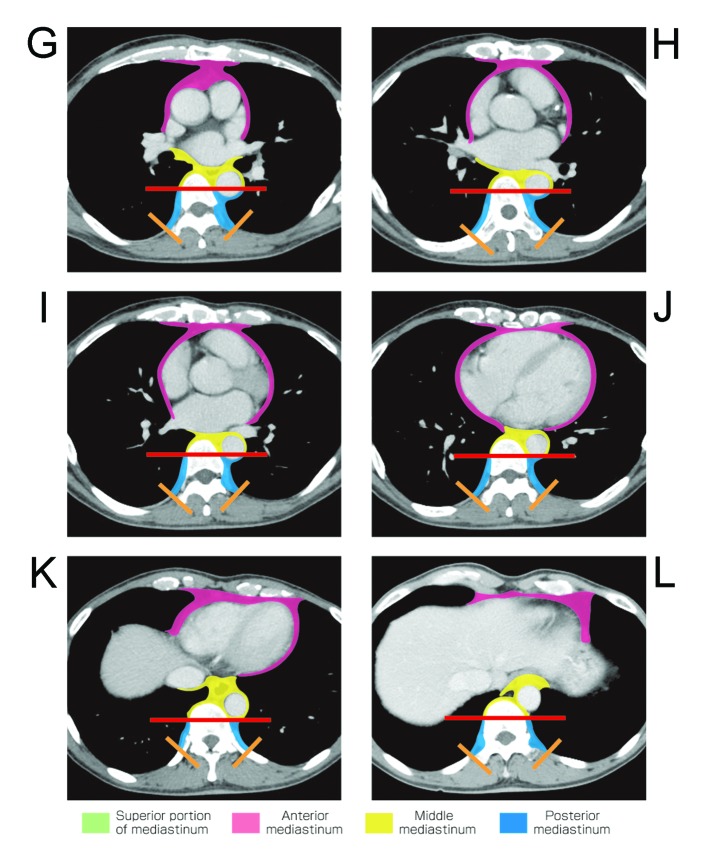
Contrast-enhanced CT images represent new proposal of mediastinal compartment classification according to the General Rules for Study of Mediastinal Tumors of the JART. (A) Thoracic inlet, (B) upper rim of clavicle, (C) sterno-clavicular joint, (D) left brachiocephalic vein across TML, (E) aortic arch, (F) tracheal carina, (G) right main pulmonary artery, (H) pulmonary trunk, (I) left atrium, (J) tricuspid valve, (K) hepatic dome of diaphragm, (L) middle of 12th thoracic vertebral body. Abbreviations: TML, tracheal mid-line; M-PBL, middle-posterior boundaryline (see Materials and methods).
